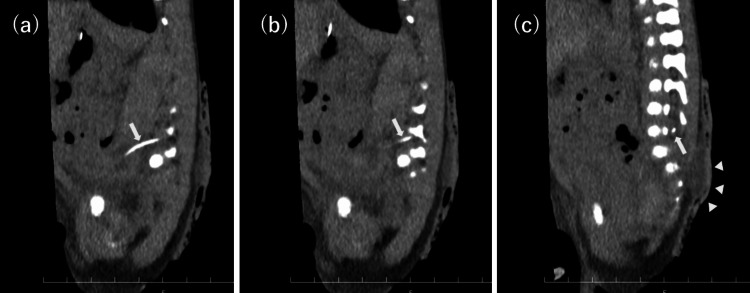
Sagittal CT image demonstrating posterior protrusion of the thecal sac at the sacral level Sagittal CT shows posterior protrusion of the thecal sac consistent with spina bifida at the sacral level (arrowhead). The peripherally inserted central catheter is also visible within the thecal sac (arrow). CT: computed tomography
